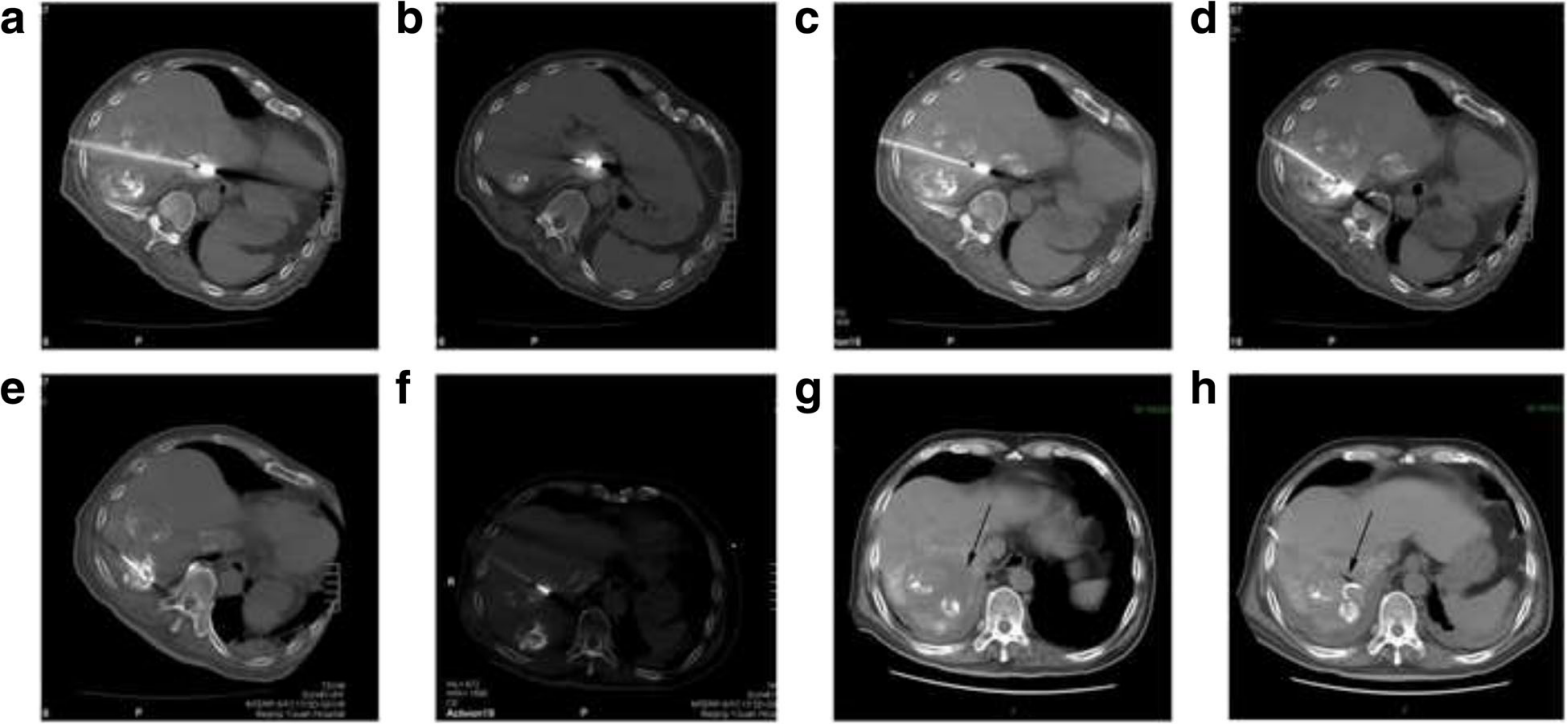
Intra-operative CT scanning and post-operative biloma. a CT scanning showed ablation of the upper part of tumor thrombus in IVC and RA. b CT scanning showed ablation of the lower part of tumor thrombus in IVC and RA. c CT scanning showed ablation of the basal part of tumor thrombus in the hepatic vein. d CT scanning showed ablation of the distal part of tumor thrombus in the hepatic vein. d CT scanning showed ablation of the distal part of tumor thrombus in the hepatic vein. e CT scanning showed ablation of the tumor in segment VII. f CT scanning showed ablation of the tumor in segment VIII. g CT scanning showed biloma which was a fluid collection in the space surrounding the necrotic tissue of the ablation zone at segment VIII (*black arrow*). h CT scanning showed significant shrinkage of biloma 10 days after drainage (*black arrow*). CT: computed tomography, RA: right atrium, IVC: inferior vena cava
